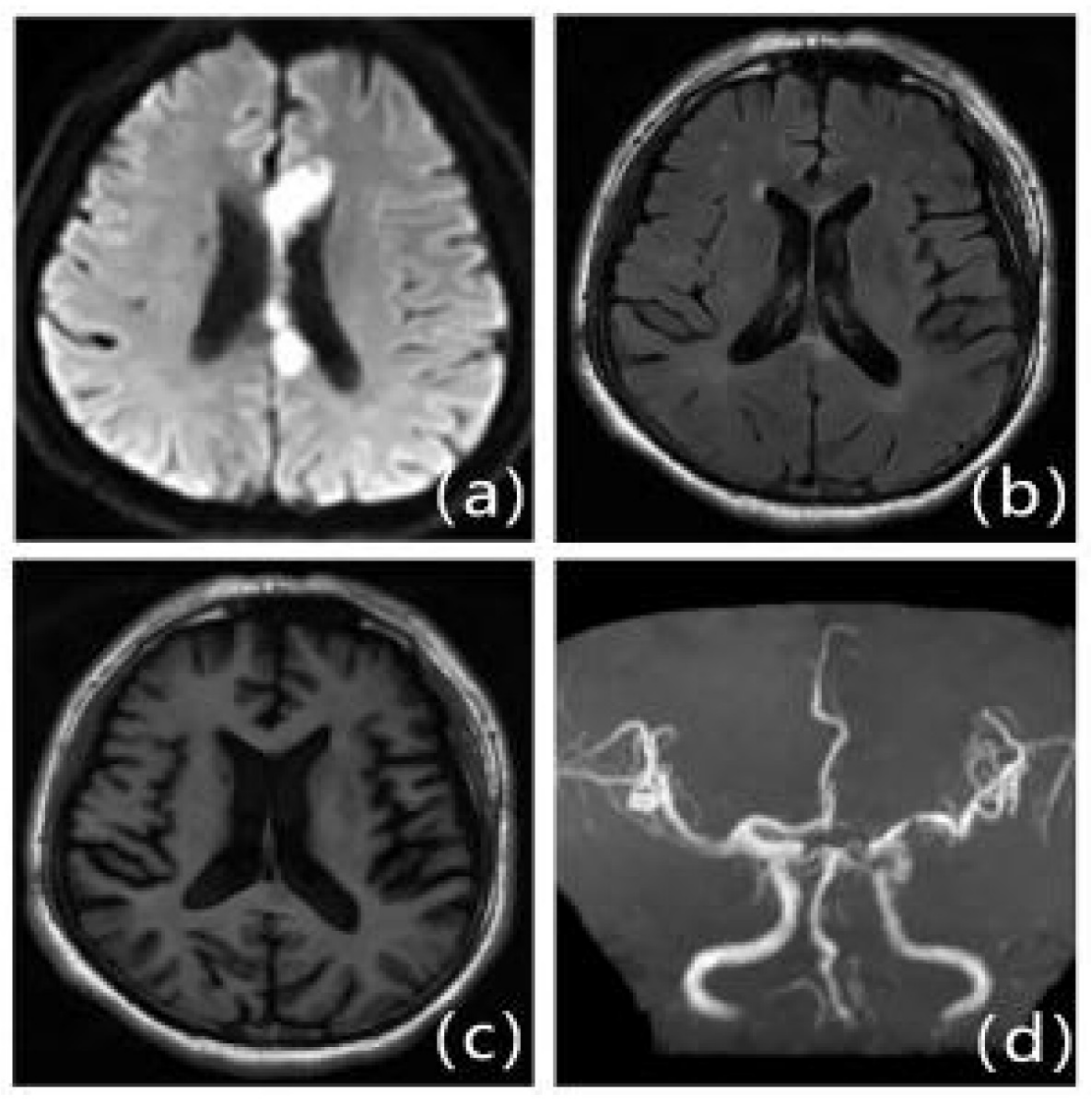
Patients with first-ever IS in ICAS with mild LA: Male, 63 years old, admitted at 15 hours of onset. A: DWI, B: T2WI-FLAIR, C: T1WI, D: MRA. DWI shows multiple lamellar fresh cerebral infarcts in the corpus callosum and left frontal lobe; MRA shows significant stenosis occlusion of the left anterior cerebral artery. T2WI-FLAIR and T1WI show mild cerebral white matter demyelination changes around the bilateral ventricles.
